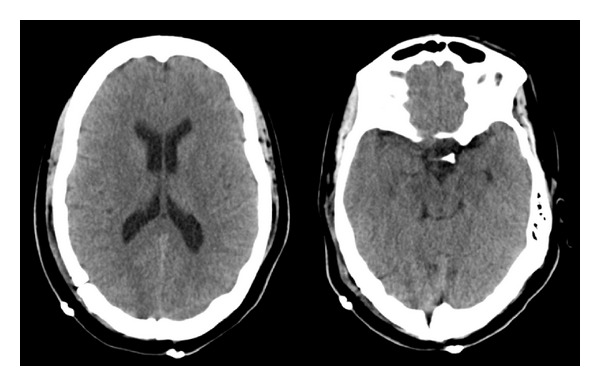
Postoperative CT scan done 2 weeks after surgery showing complete resolution of pneumocephalus.
